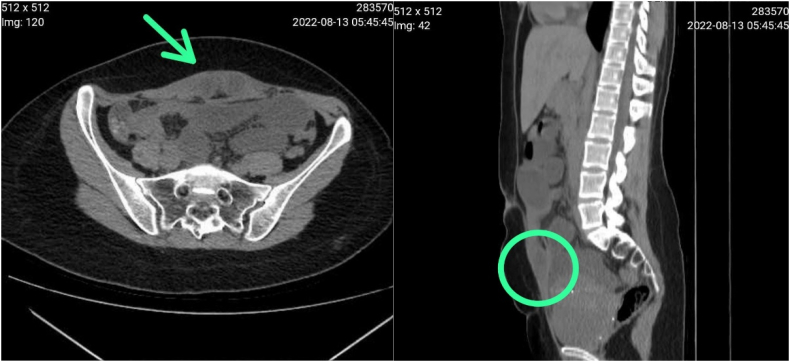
Computed tomography scan showing posterior rectus sheath hernia.
